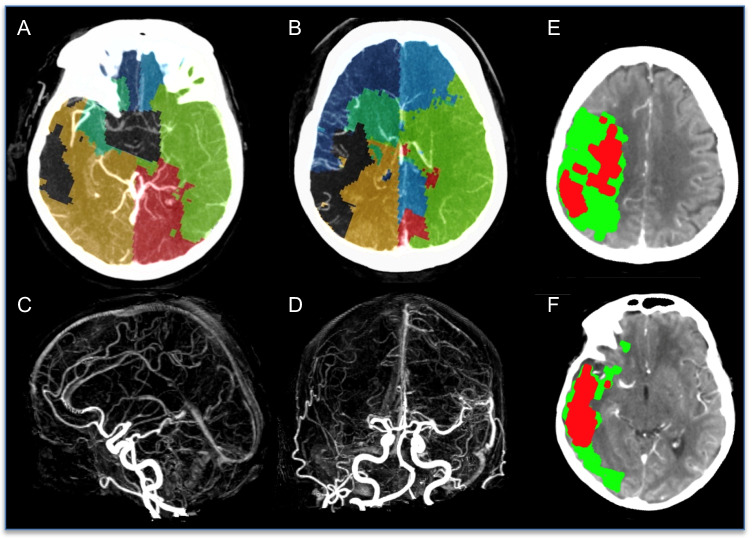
Right MCA occlusion with poor collaterals. Occluded proximal right M1. A, B Axial slices of CTA with VTM overlay. C, D MIP CTA demonstrates occlusion of the right MCA. E, F CT perfusion summary (core/penumbra map) slices. In contrast to the patient in Fig. 2, here is a patient with a poor collateral circulation that demonstrates a minimal supply to the right MCA region (dark green) from the ipsilateral MCA with some compensatory increase of both ACA and PCA supply on the ipsilateral side. Affected hemisphere ACA and PCA territories have not expanded to the same extent as seen in Fig. 2, with smaller ipsilateral ACA/PCA territories on VTM. Given very delayed regions of contrast flow due to this poor retrograde flow from ACA and PCA within the (normally) right MCA supplied territory, there are areas with an absence of territory marking (grey). The penumbral region on CT perfusion extends to the entirety of the right MCA territory (green), again reflecting the marked delay in contrast transit (delay time > 3 s) due to poor collateral flow. On CTP there is 213 ml of penumbra (green) and 78 ml of perfusion core (red). The substantial perfusion core is present in the watershed zones (boundary of ACA/PCA territories) on the VTM map, and is very similar to the unmasked (grey) area on the VTM map, reflecting profound ischemia and severe delay
